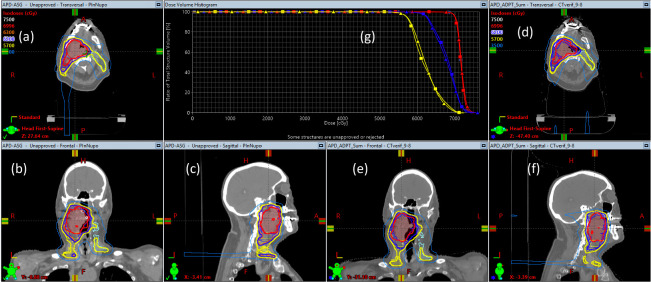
An example of adaptive planning restoring the planned dose distribution. (a-c) Dose distribution of the original plan. (d-f) Dose distribution of the adaptive plan. The dose distribution in (d), (e), and (f) is the summation of the original plan for 23 fractions and adaptive plan for 10 fractions showing on the CT at verification. (g) Dose-volume histograms for the original plan (square) and summation of the original plan and adaptive plan (triangle). Abbreviation: CT, computed tomography.
